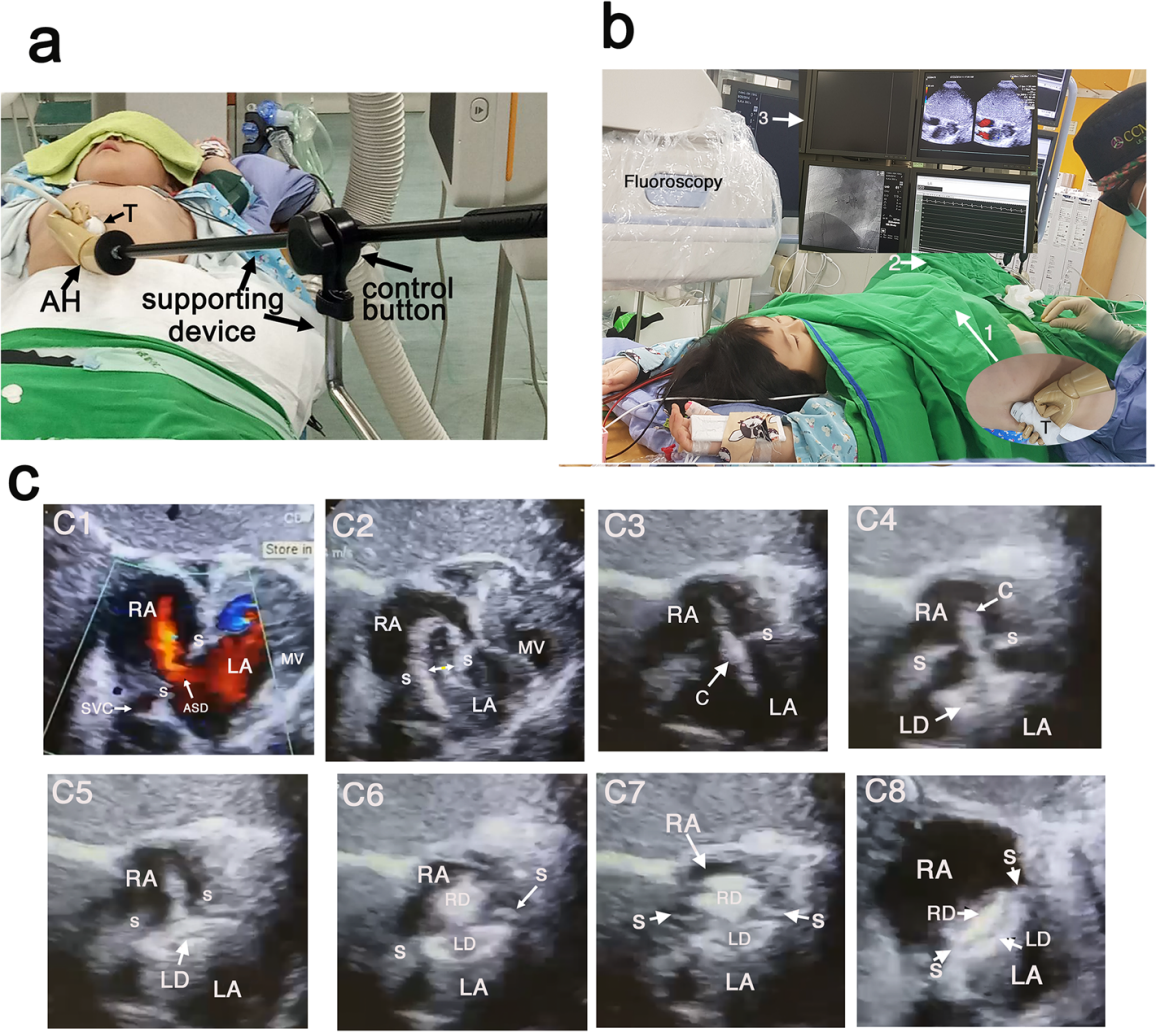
a The AH-TTE monitoring system consists of an artificial hand (AH) and a supporting device. The transthoracic transducer is held by the AH and secured in place at the subcostal area by locking the control button to obtain subcostal views and provide real-time TTE imaging during the entire procedure. b A 4-year-old girl during ASD closure using AH-TTE monitoring system. The AH grasping the transducer was placed in the subcostal area (arrow 1: AH; arrow 2: supporting device) and the procedure was performed after the area was covered with an aseptic cloth. c The correspondent real-time TTE imagings (arrow 3 in B) were demonstrated during ASD closure procedure. C1: An ASD (11.5 mm in size) with a left-to-right shunt. C2: The waist length of the ASD was 11.3 mm (denoted by the two-way arrow) as determined by balloon sizing. C3: The correct passing of the catheter through the defect. C4: The left disc (LD) was deployed in the LA. C5: The LD was pulled back against the atrial septum. C6: The RD was opened in the RA. C7: The RD was deployed in the RA. C8: An 11-mm Amplatzer™ Septal Occluder was successfully implanted and positioned in the atrial septum without requiring shunting. Abbreviations: AH, artificial hand; R, right; L, left; O, occluder; TTE, transthoracic echocardiography; RD, right disc; LD, left disc; RA, right atrium; LA, left atrium; T, transducer; S, septum
